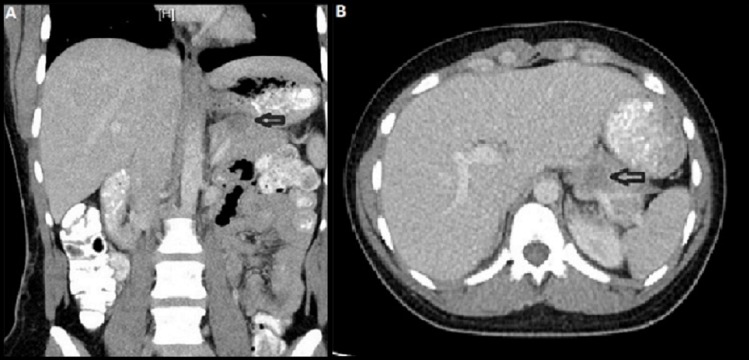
Computed tomography (CT) scan of the abdomen (A): Coronal view of abdominal CT scan showing a 2 x 2 cm pancreatic lesion (arrow); (B): Axial view of abdominal CT scan showing the pancreatic lesion (arrow)
